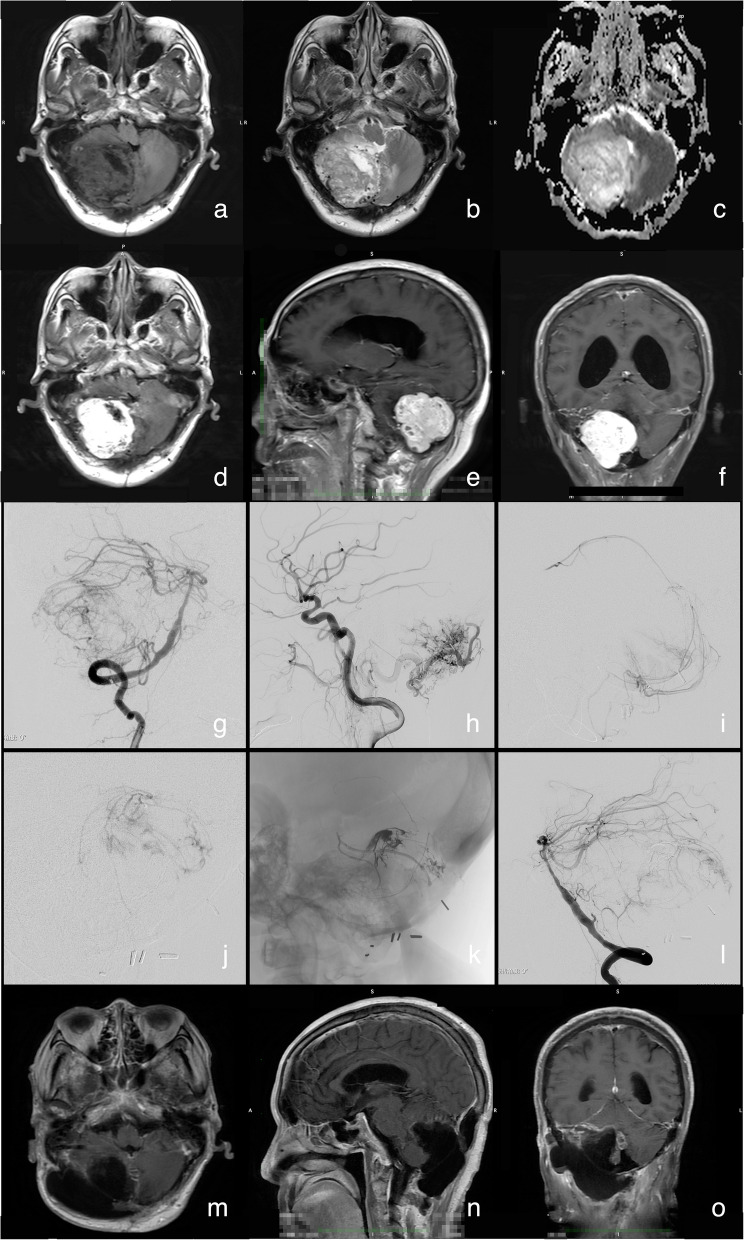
a and b MRI revealed postoperative changes in the posterior cranial fossa, with a mass-like ring-shaped signal-occupying lesion visible in the right cerebellar hemisphere. The borders of the lesion are ill-defined, and multiple vascular flow voids are observed around the white matter. The fourth ventricle is compressed and deformed, and the supratentorial ventricles are dilated. c Diffusion-weighted imaging revealed no significant diffusion restriction in the lesion. d, e In contrast imaging, the lesion demonstrated marked heterogeneous enhancement. g and h DSA revealed a hypervascular tumor in the right cerebellar hemisphere supplied by the right posterior inferior cerebellar artery, right superior cerebellar artery, right anterior inferior cerebellar artery, and dural branch of the right occipital artery. The tumor is drained by the superficial veins of the cerebellar hemisphere. i An angiogram via the microcatheter, which had superselected into the distal end of the posteroinferior cerebellar artery, verified the feeding of the tumor before embolization. j Microcatheter angiogram of the distal end of the anterior inferior cerebellar artery, which verified the supply of the tumor before embolization. k Embolic agent cast in the pedicles of intracranial major arterial feeders and capsule vessels. l The endovascular procedure achieved partial obliteration of the tumoral arterial feeders, leaving tenuous feeders from the anterior inferior cerebellar artery and occipital artery to be controlled microsurgically. m, n, o Follow-up magnetic resonance imaging at 1 month revealed gross-total resection of the tumor, resulting in postoperative subcutaneous effusion at the surgical site
